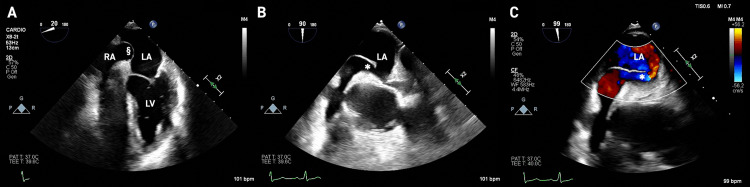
Patient's transesophageal echocardiogram The transesophageal echocardiogram revealed a patent foramen ovale. The contrast test with agitated saline confirmed the existence of an intracardiac right-left shunt. RA: right atrium, LA: left atrium, LV: left ventricle, *:* *foramen ovale
